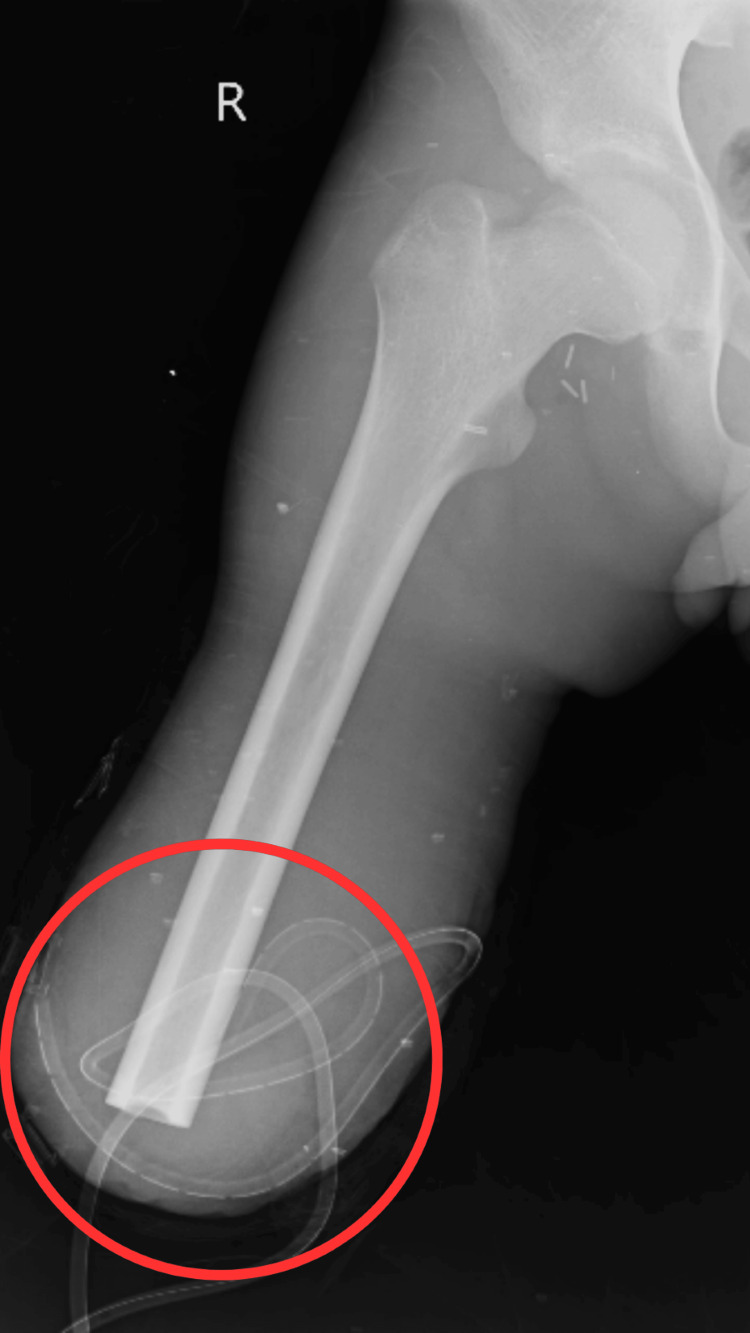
X-ray anteroposterior view The red circle represents the right amputated lower limb
